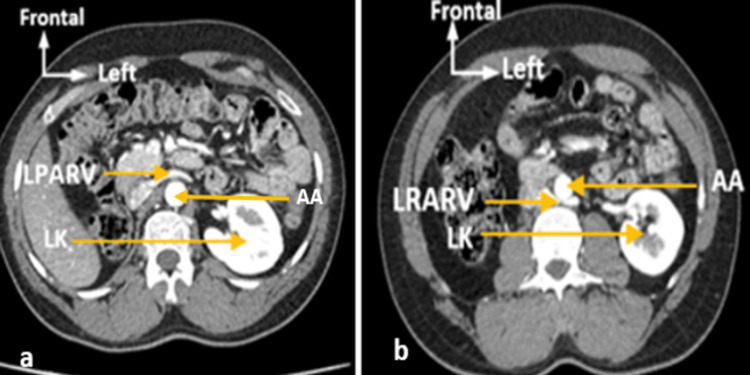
Axial 2D CT section showing (a) Left pre-aortic renal vein (LPARV) and (b) Left retro-aortic renal vein (LRARV). AA: Abdominal aorta; LK: left kidney
